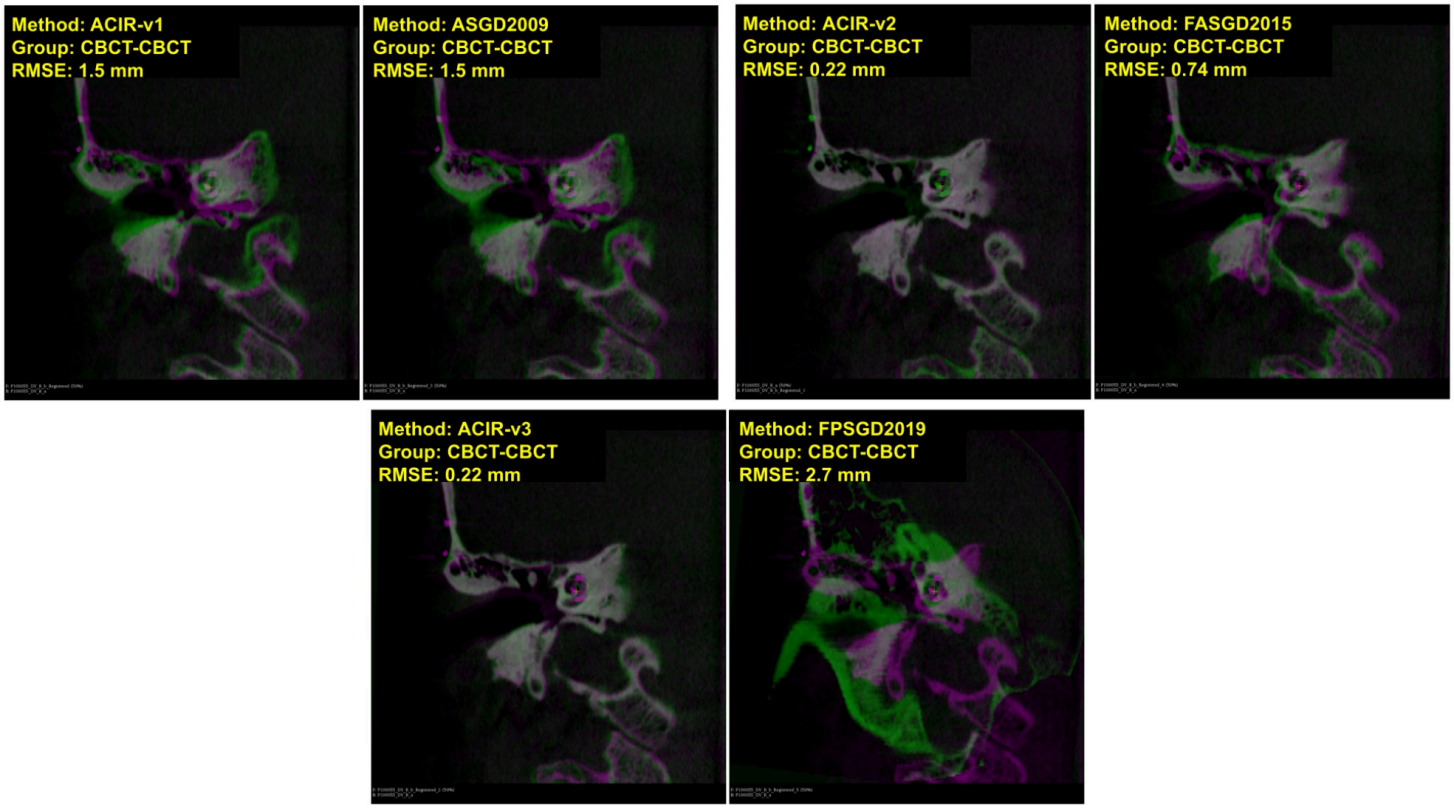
Sample results of registration CBCT to CBCT (coronal views). The figure shows image types, methods, and RMSE information. Magenta represents the fixed image and green represents the moving image.
